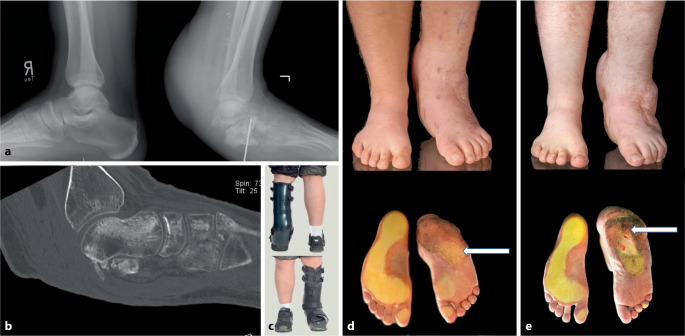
a–d Erstvorstellung 6 Monate nach dem Unfall mit 5 Voroperationen, zuletzt mit M.-latissimus-dorsi-Lappen a, b Seitliche Belastungsaufnahmen zeigen die subtotale Kalkanektomie, eine Tibiainklination mit verbliebenem K‑Draht. Das CT (b) zeigt eine fragliche avaskuläre Nekrose bzw. schwere Demineralisierung des Talus, c Spezialorthese, d Spiegeltisch-Aufnahme (präoperativ) mit fehlender knöcherner Ferse, Mittfußlastaufnahme (*Pfeil*), e 8 Monate nach Kalkanogenese mit erkennbarer Rückfußvergrößerung und Dorsalisierung der Lastaufnahme (*Pfeil*)
